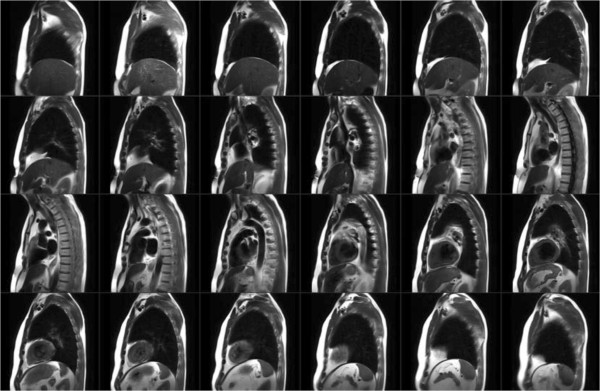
Example images using free-breathing magnetic resonance imaging in sagittal plane employing the HASTE pulse sequence. Adipose tissue demonstrates high signal relative to all other tissues.
